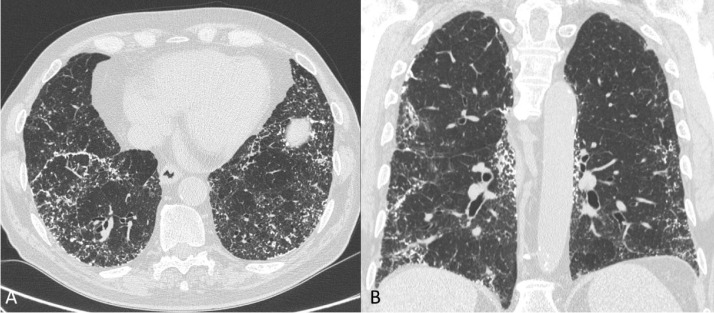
A - B. Follow-up HRCT showing an increase in extent of pulmonary calcifications (axial and coronal reconstructions in A and B, respectively).
